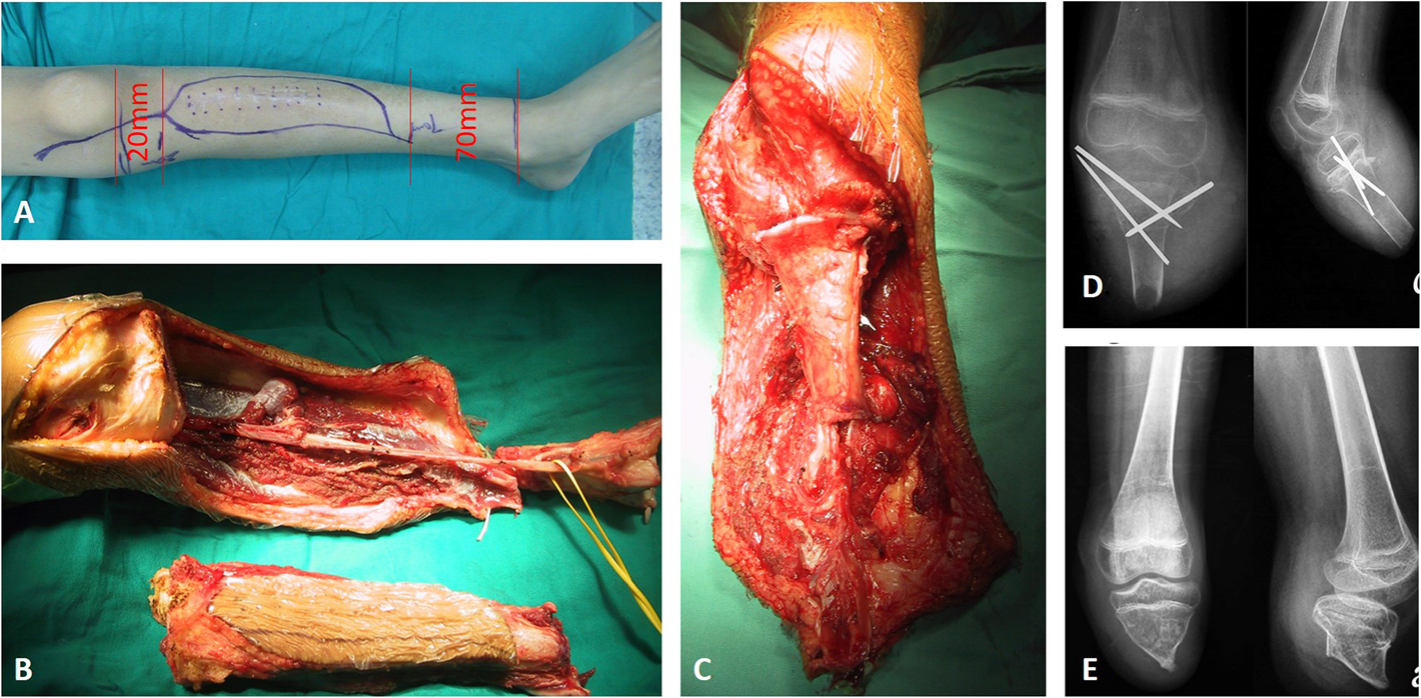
Radical removal of the tumor and preservation of the blood supply on the distal tibia. a Incision was marked preoperatively. b Tumor and surrounding tissues were resected 2 cm below the knee. The tumor and surrounding tissues were removed. c Osteosynthesis with Kirschner wires and preservation of the tibial epiphysis. d X-ray performed 1 month after the operation. e X-ray performed 2 years after the operation
